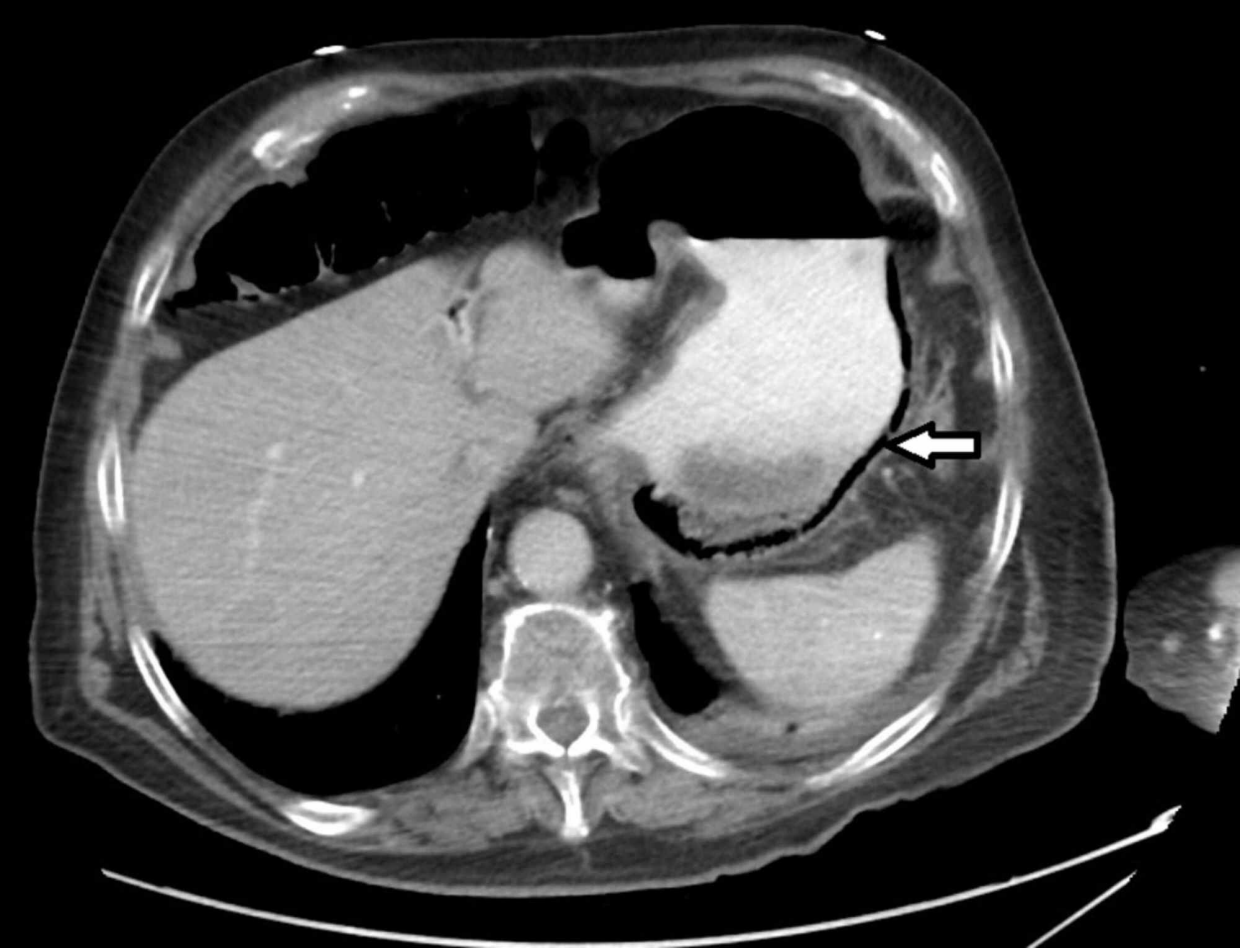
Computed tomography of the abdomen and pelvis showing gastric wall thickening along with circumferential gas formation (arrow).
